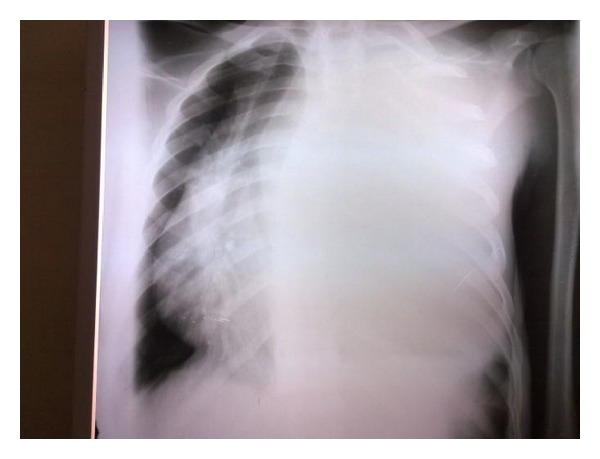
Chest radiograph showing homogenous opacification of the entire left hemithorax with marked mediastinal shift to the right.
